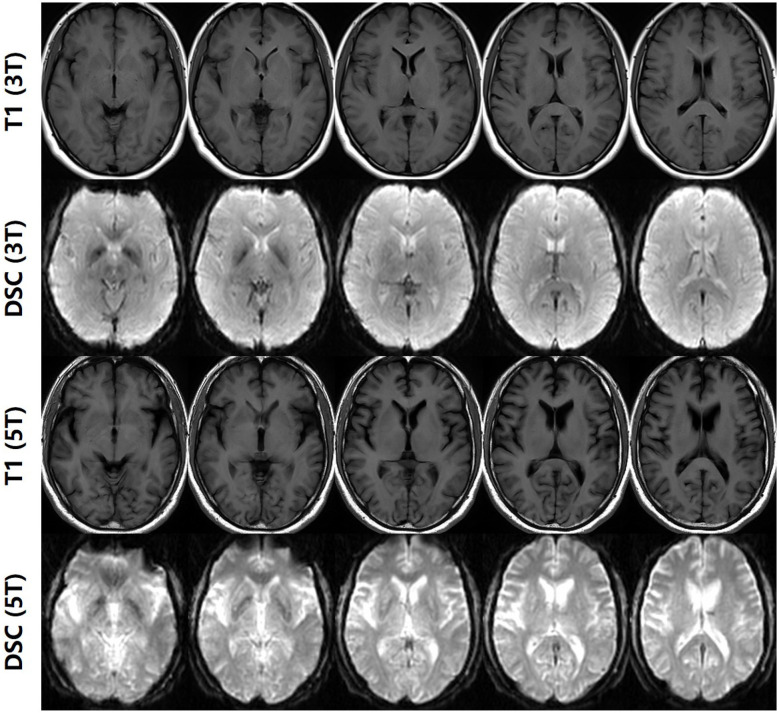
Demonstration of T1-weighted image and DSC-MRI image from 3T and 5T.
